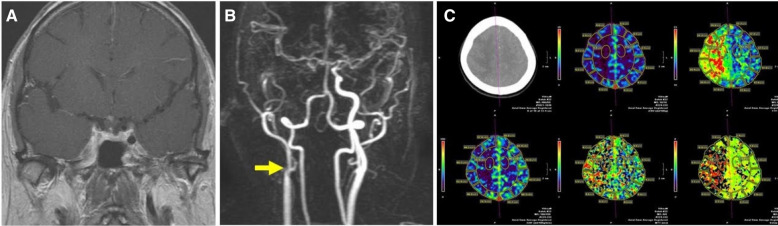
A Pre-operative brain magnetic resonance imaging showing right side cavernous sinus thrombosis with internal carotid artery occlusion. B Magnetic resonance angiography demonstrating retrograde occlusion at the common carotid bifurcation (*arrow*). C Computed tomography perfusion showing impaired perfusion in the right middle cerebral artery territory
